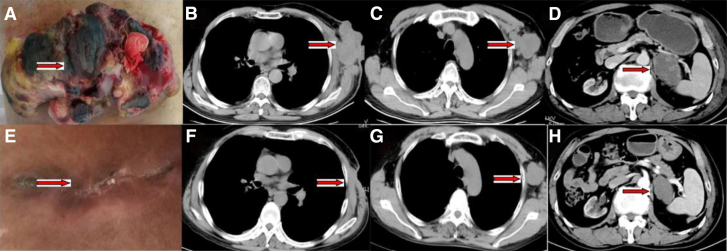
Initial CT scans: (A) Metastatic tumor measuring 13.0 × 6.0 cm located on the left thoracic wall, exhibiting necrotic hemorrhage on the surface; (B) subcutaneous soft-tissue metastasis of the left thoracic wall under CT; (C) left axillary lymph node metastatic tumor with a maximum diameter of 6.4 cm; (D) left adrenal metastatic tumor with a maximum diameter of 6.5 cm. After 6 cycles of medication: (E) Subcutaneous soft-tissue metastasis of the left thoracic wall has disappeared and the superficial skin began to heal; (F) subcutaneous soft-tissue metastasis of the left thoracic wall has disappeared under CT; (G) left axillary lymph node metastatic tumor with a maximum diameter of 3.7 cm; (H) left adrenal metastatic tumor with a maximum diameter of 5.1 cm. CT = computer tomography.
